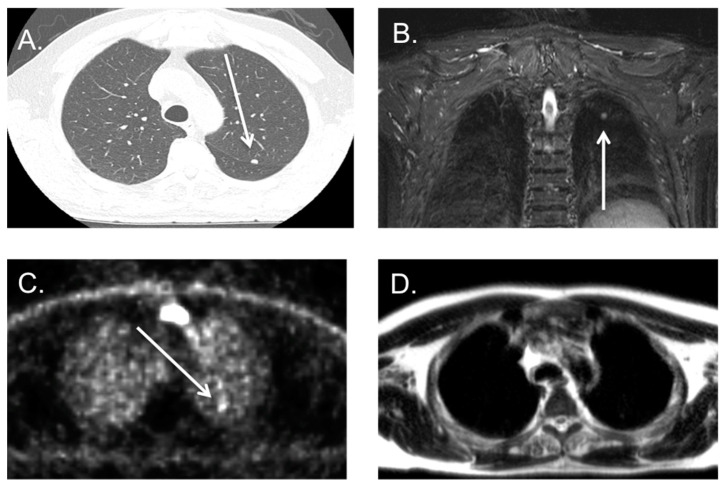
Patient with lung metastasis on both a CT and PSMA PET-MRI scan; [^68^Ga]-PSMA PET-MRI and low dose CT in an 84-year-old male showing a nodule of 6 mm in the left upper lobe on CT, see white arrow (A). The nodule is visible on the T2 weighted fat suppressed (STIR) images, see white arrow (B) and intense PSMA-avid, see white arrow, (C), but hardly visible on the axial T2 sequence (D).
